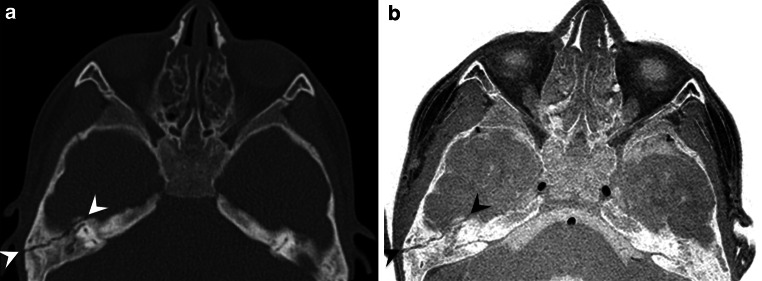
Axial reformation of a CT (a) and inverted 3D T1-weighted spoiled gradient echo sequence (b) of the skull of a 6-year-old patient with a non-displaced fracture of the right temporal bone (*arrows*)
